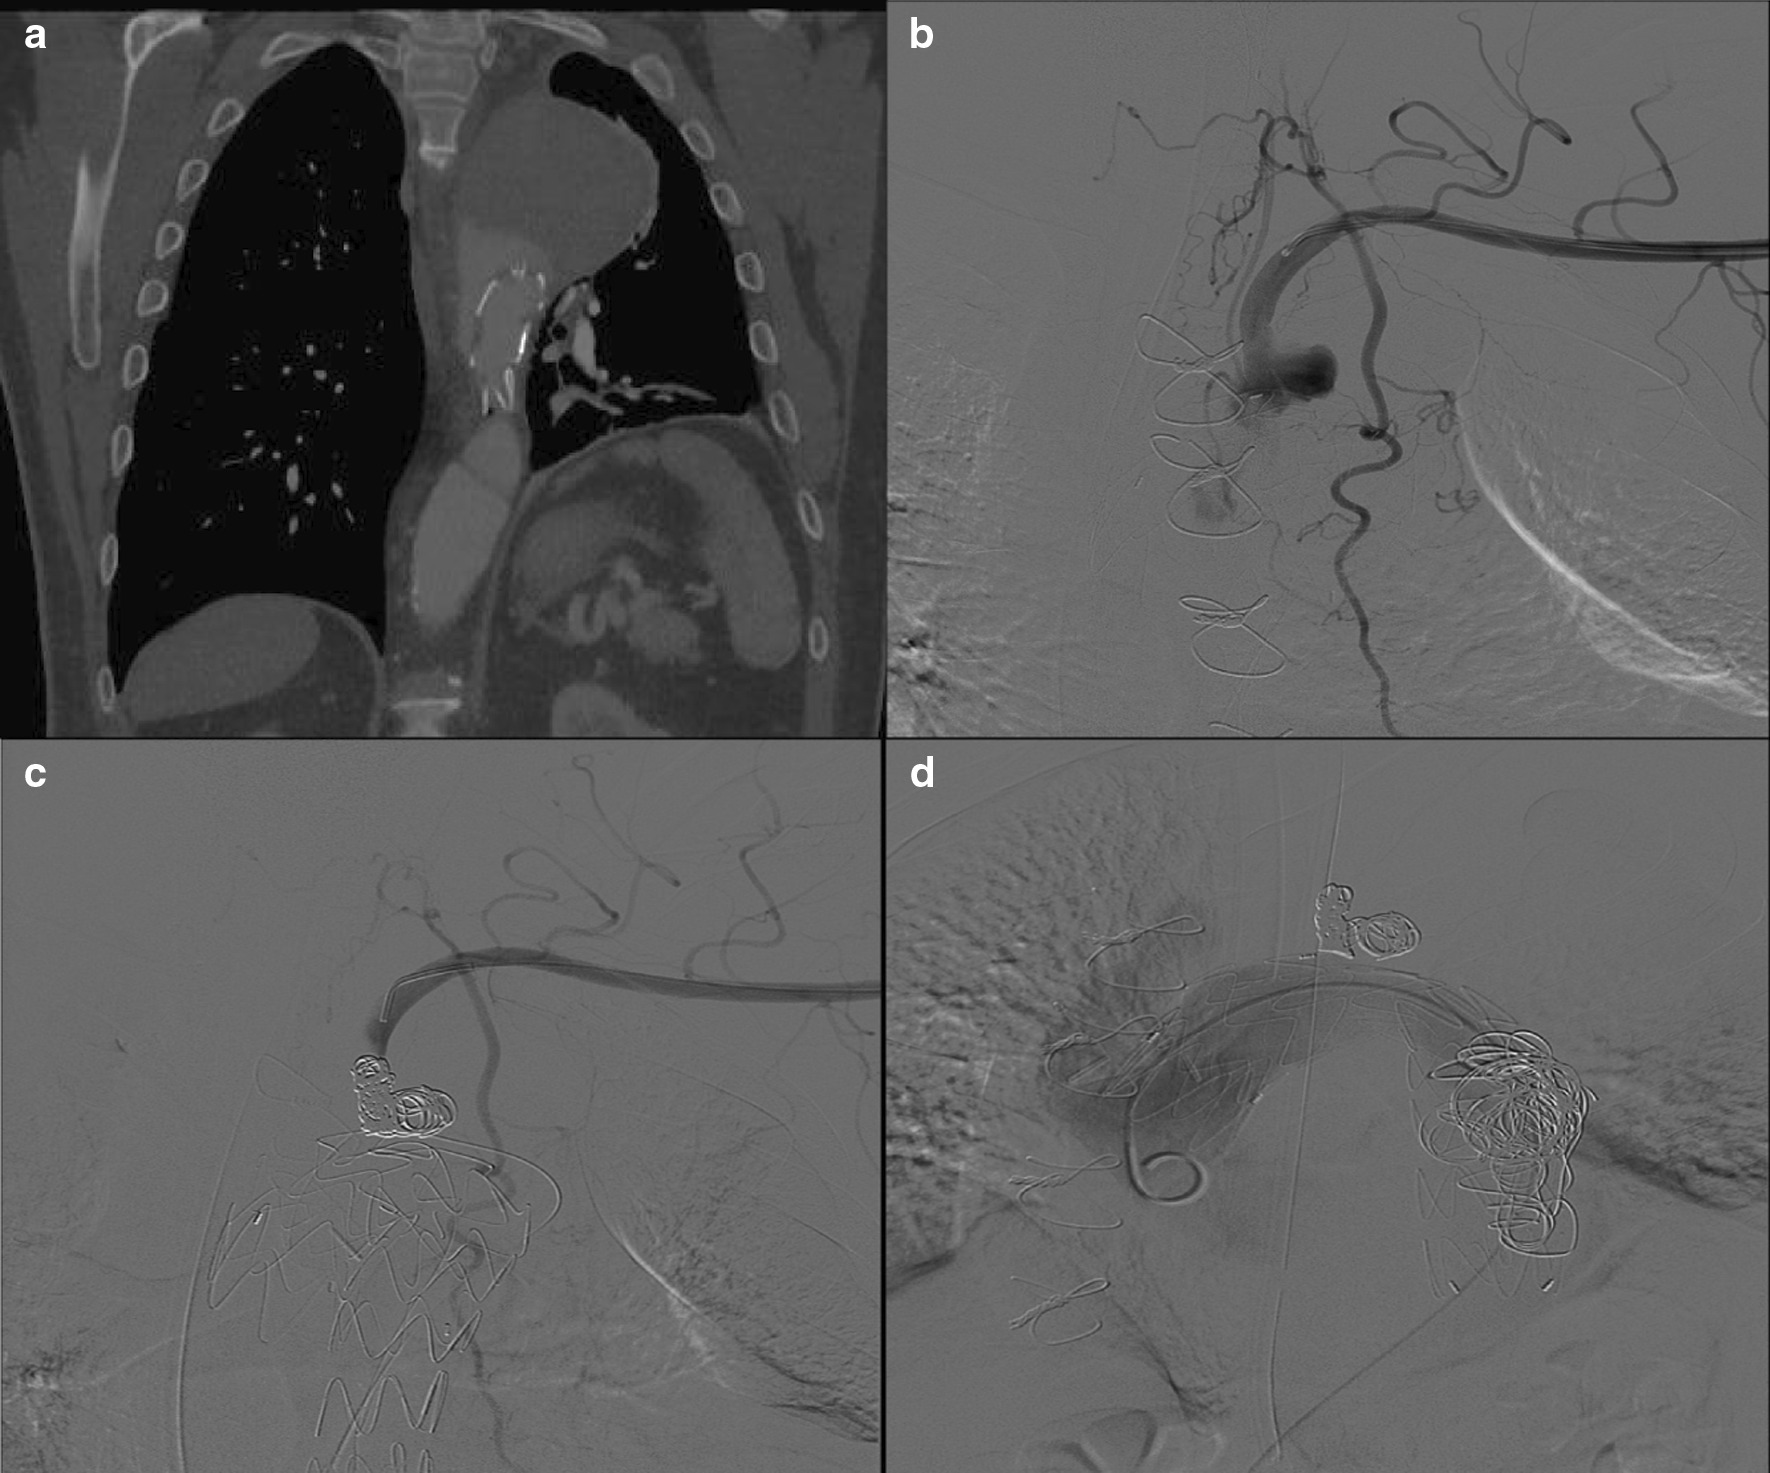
a Post-thoracic endovascular aortic repair (TEVAR) computed tomography angiography demonstrating an endoleak around the TEVAR, likely type II from multiple thoracic perforators and left subclavian artery (LSA). b Angiogram from left brachial catheter demonstrating type II endoleak from the LSA filling the aneurysm sac and multiple collaterals. c Coil embolization of the LSA with subsequent absent filling of the aneurysm sac. d Angiogram at the level of the TEVAR after coil embolization of the false lumen with no evidence of endoleak
